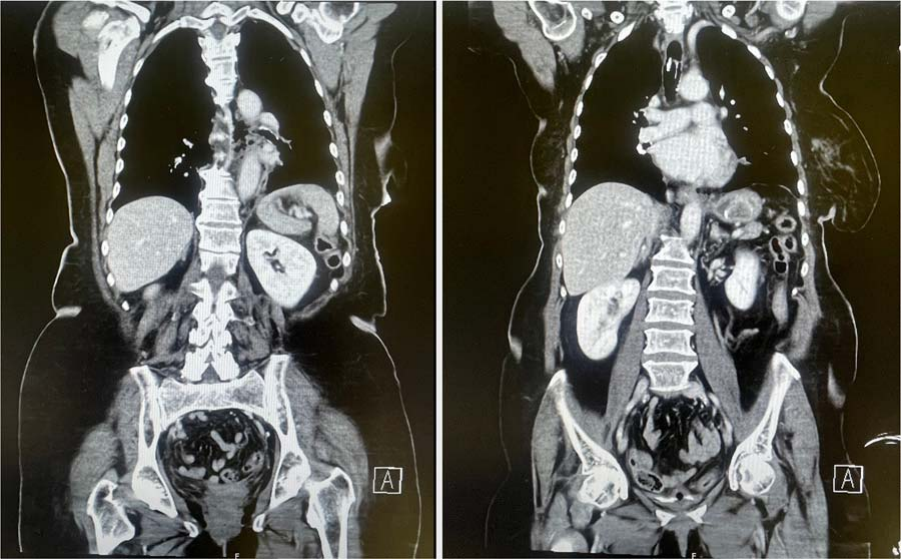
CT scan showing mediastinal air consistent with esophageal perforation (left) and a hyperdense structure in the stomach corresponding to the gallstone within the Dormia basket (right).
